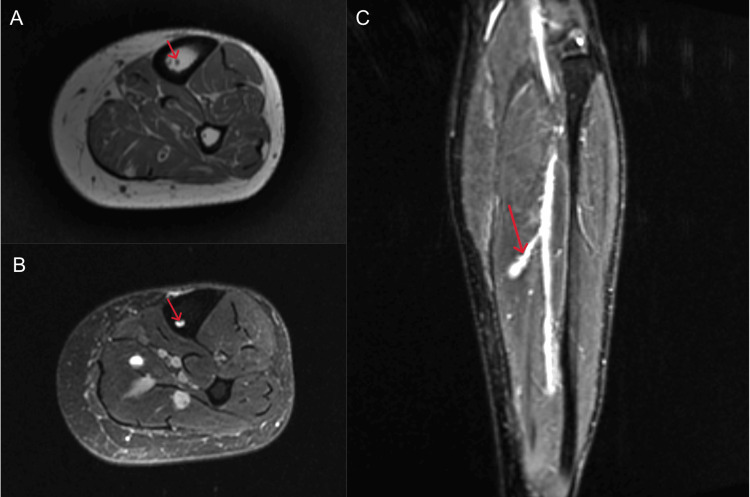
MRI of the left tibia and fibula (panel A: axial T1 fat-saturated sequence, panel B: axial T2 fat-saturated sequence, and panel C: coronal short tau inversion recovery sequence) showing a prominent intraosseous varix at the mid tibial level
